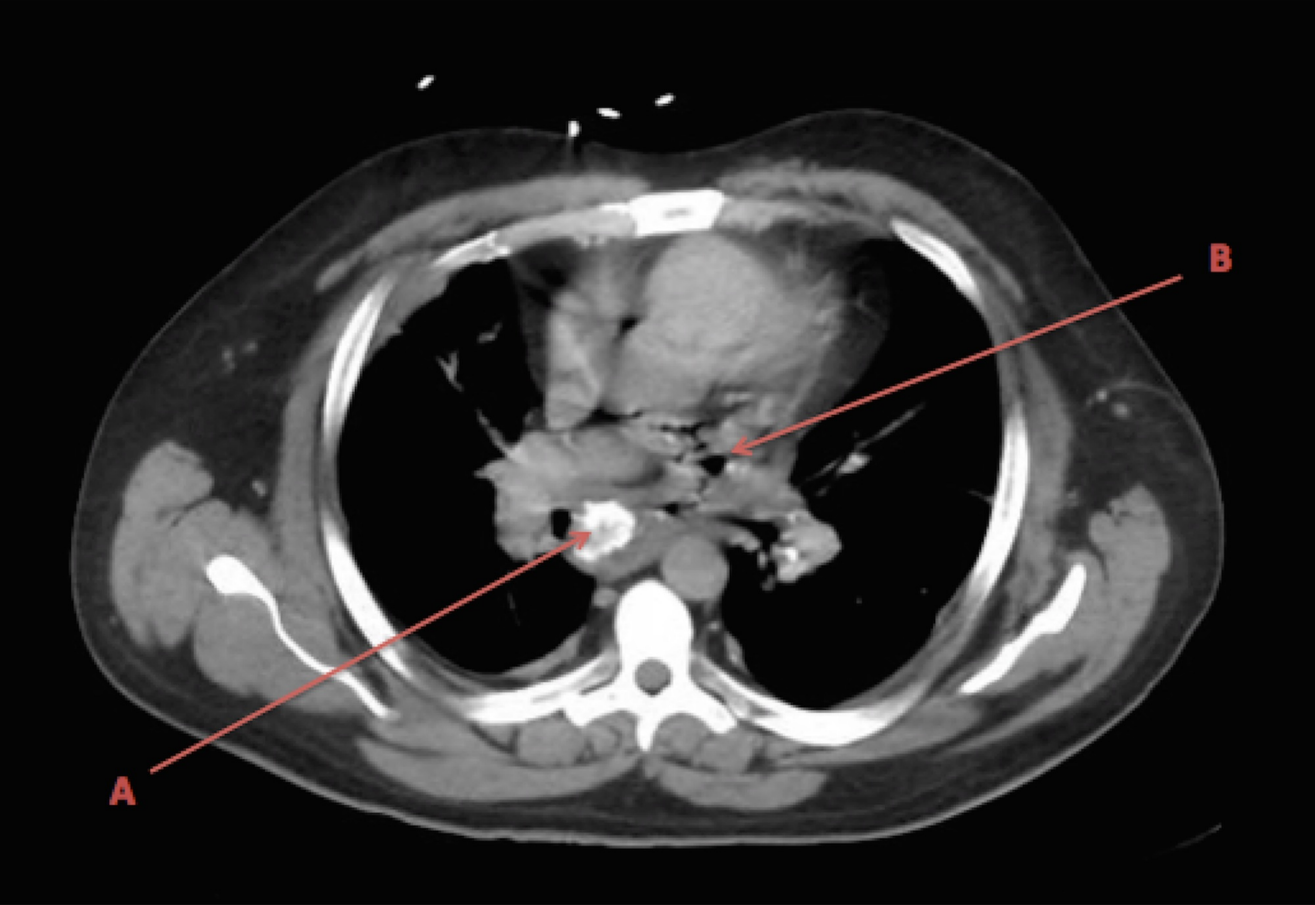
CT demonstrating calcified lymphnode (A) and pneumomediastinum (B) due to the fistula
